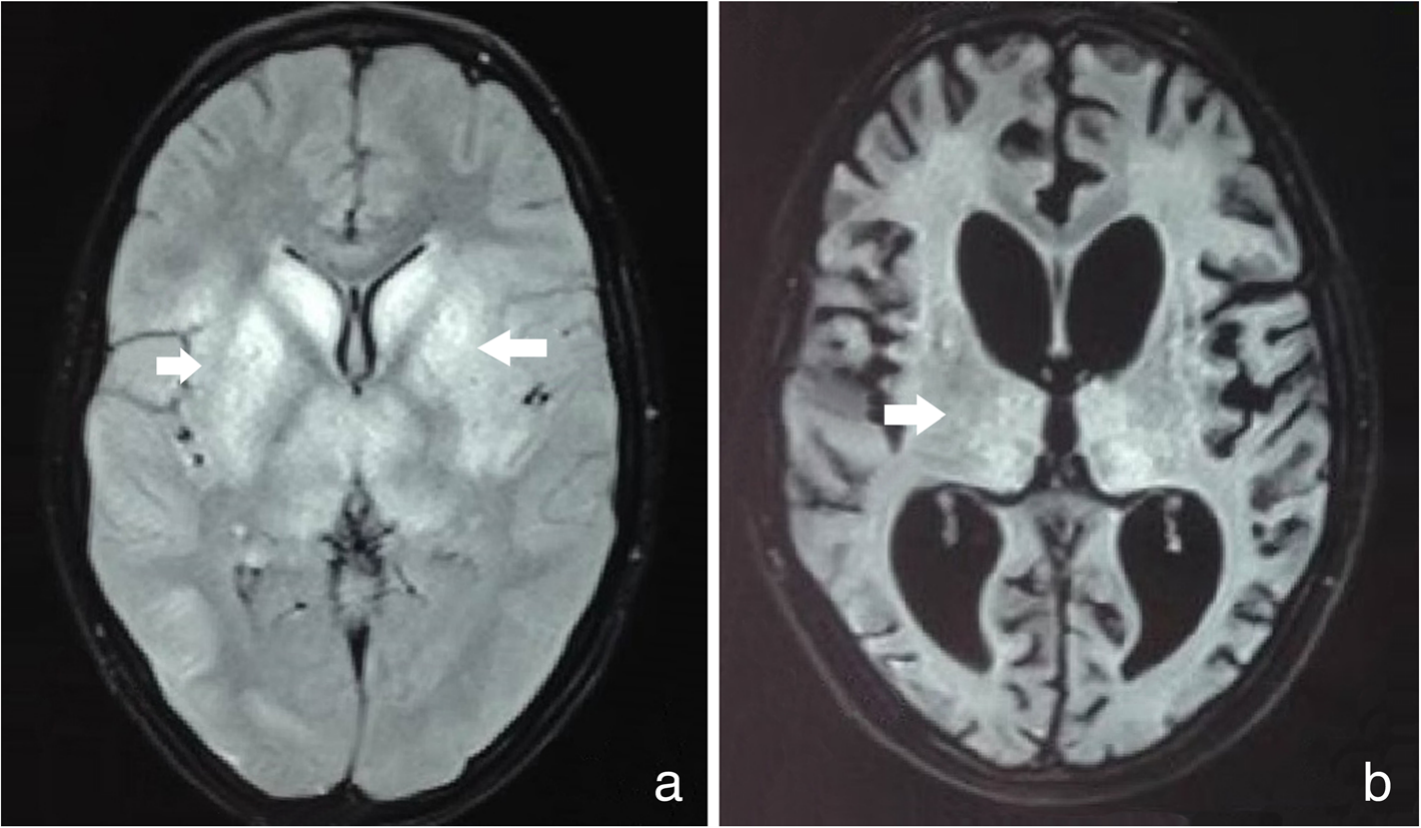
MRI images (FLAIR) performed one month after the patient was bitten; hyperintensities in the basal ganglia (*white arrows*), thalamus, pons, and medulla (a) and brain atrophy (b) are shown
